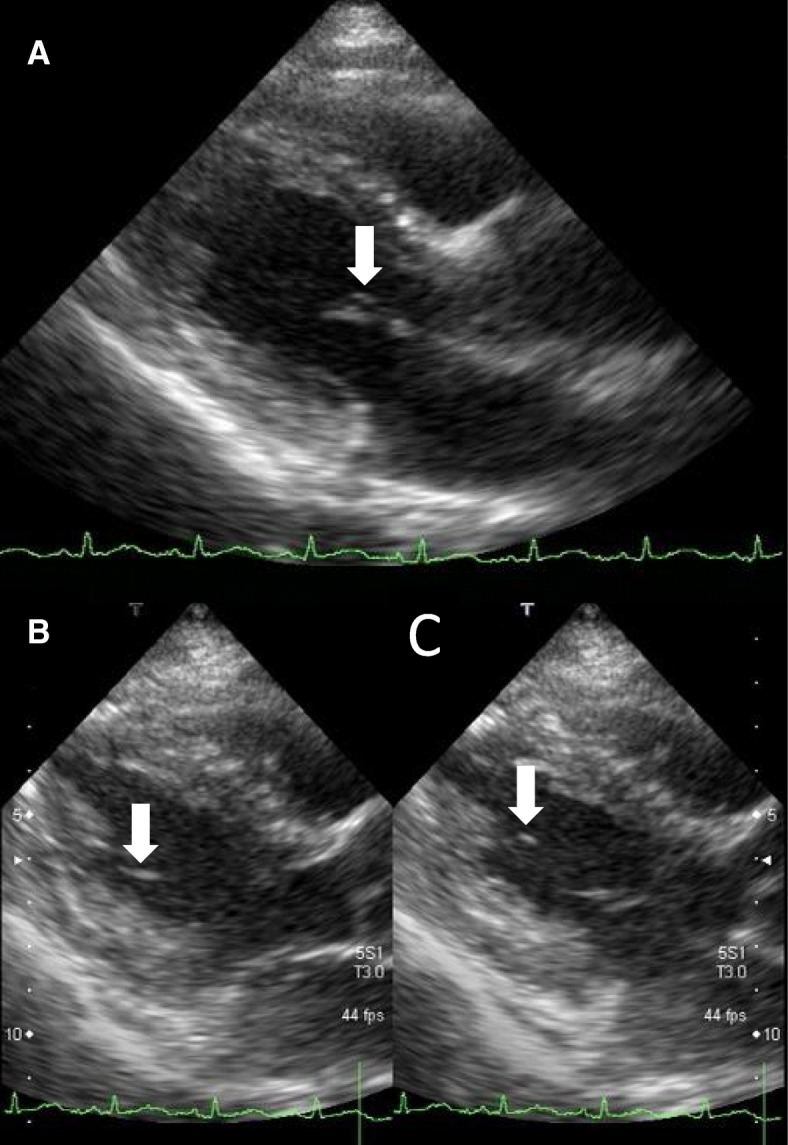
Transthoracic echocardiography images on day 2. a: A parasternal long-axis tomogram demonstrated a vegetation attached to the anterior leaflet of the mitral valve (4 × 1 mm, white arrow). b, c: Parasternal long-axis tomograms at (b) presystole and (c) end-diastole (white arrows.) A mobile and elongated vegetation (10 × 2 mm) was attached to the chorda tendinea of the mitral valve
